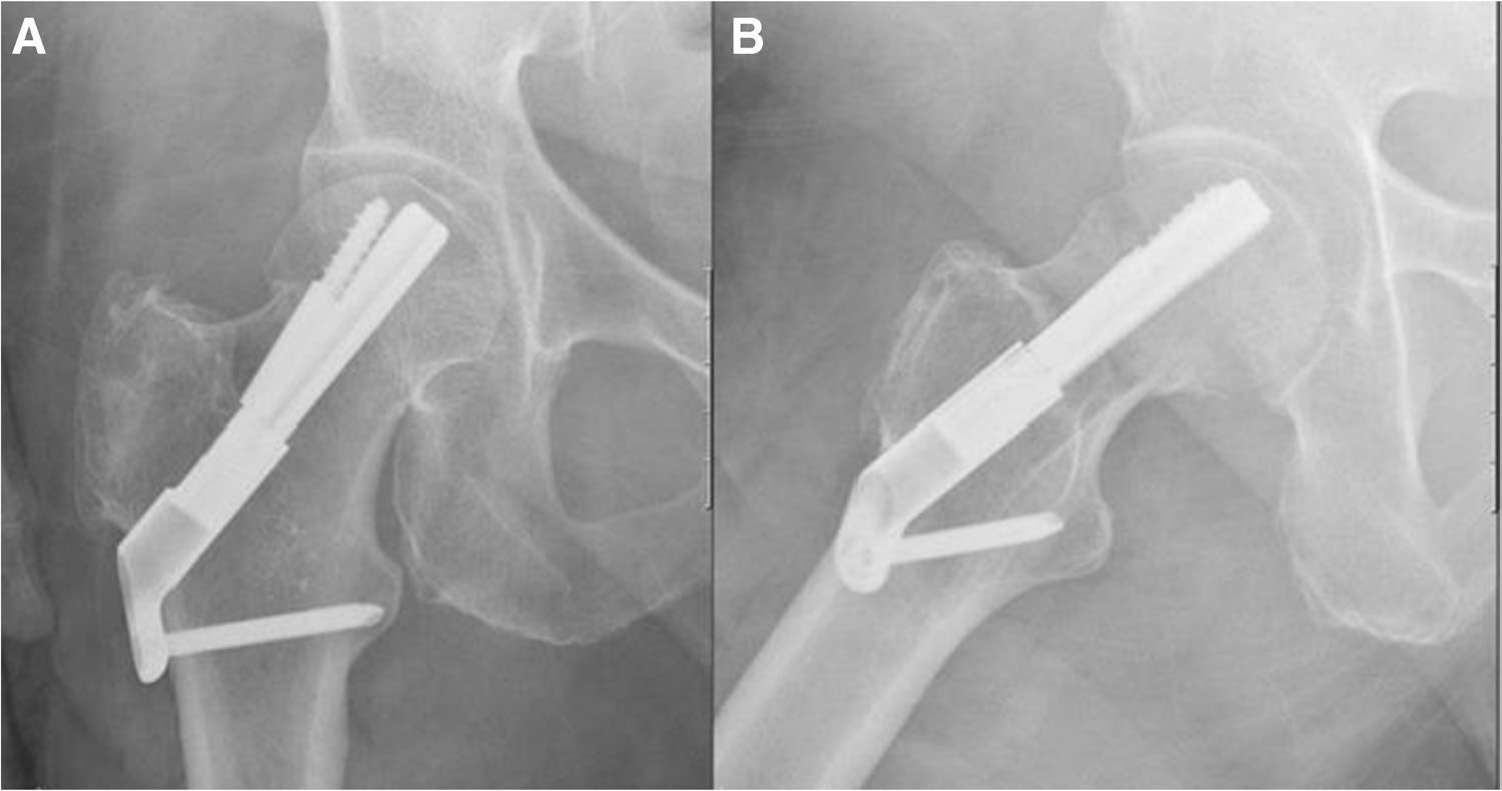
A, B AP and lateral radiographs postoperative day 1, demonstrating fixation of the fracture with FNS
